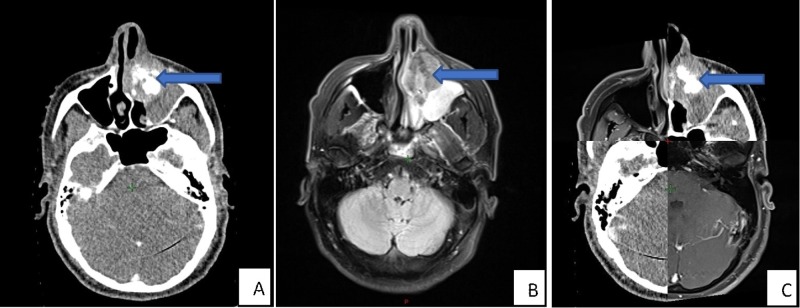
Pre-treatment imaging A – CT: computed tomography, B – MRI: magnetic resonance imaging, and C – CT/MRI fusion scans showing the left primary sino-nasal neuroendocrine carcinoma (PSNNEC) by blue arrowheads
